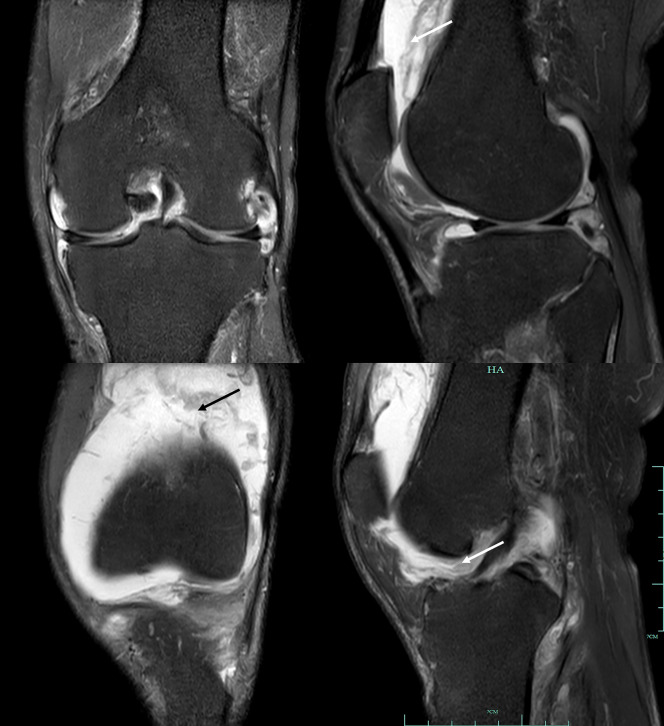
Magnetic resonance images of the patient. White and black arrows indicate cloud-like material within the joint cavity.
